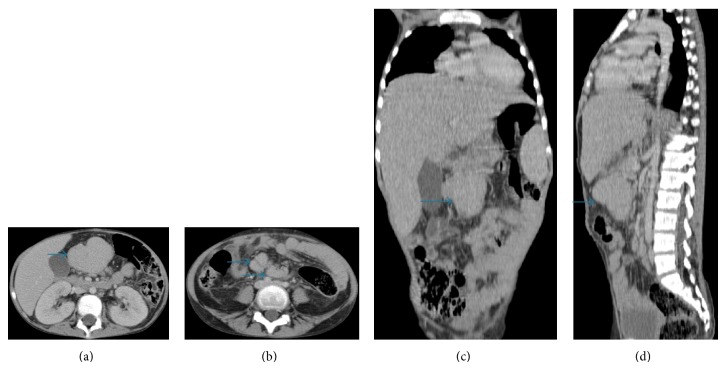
Thoracoabdominal-pelvic computed tomography injected in axial sections (a and b) with coronal (c) and sagittal (d) reconstructions demonstrating multiple adenomegalies and a mesenteric soft tissue mass.
